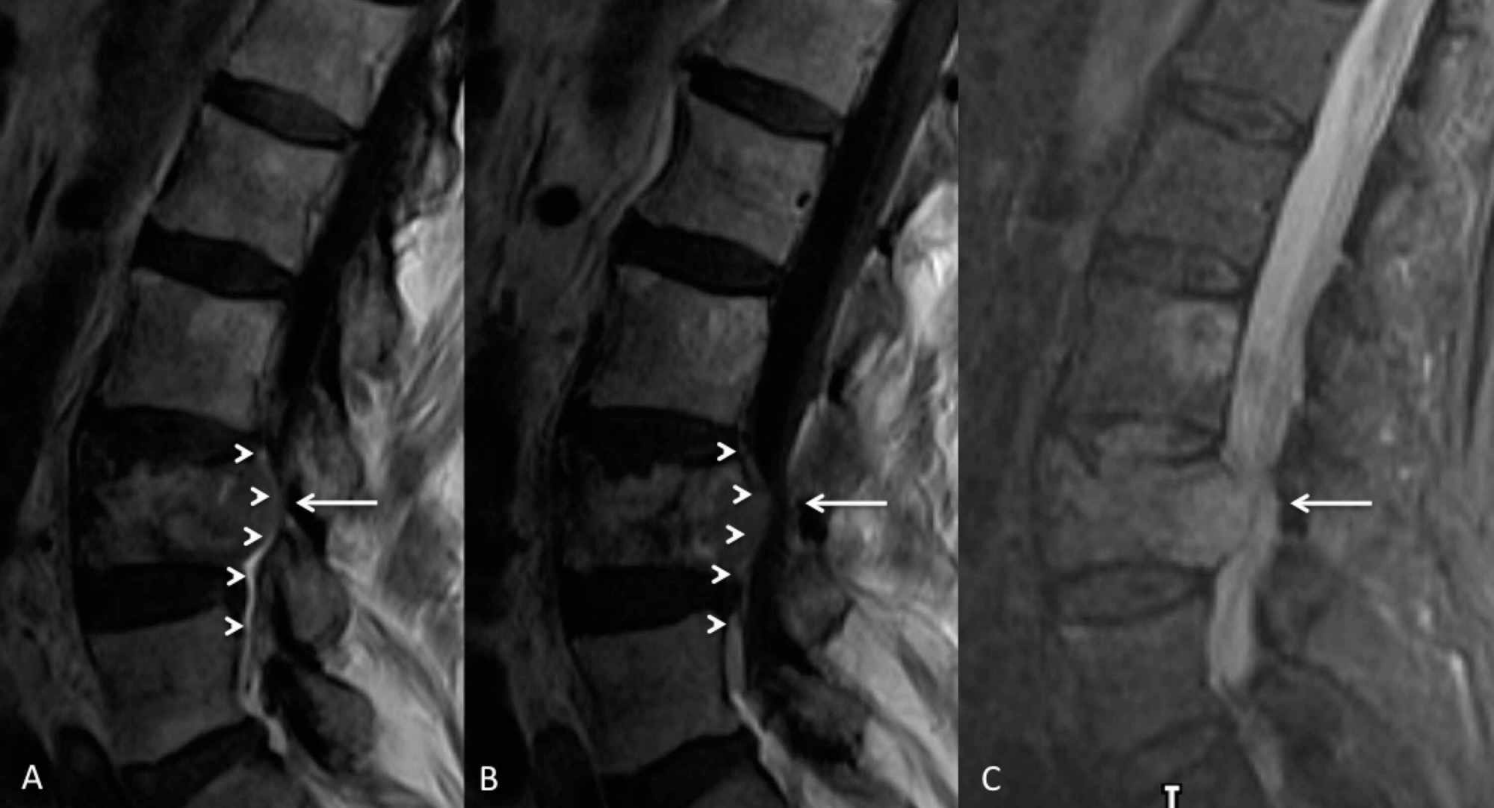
Pre-treatment parasagittal contrast-enhanced T1-weighted image (A), T1-weighted (B) and STIR-weighted (C) acquisitions demonstrating an expansile metastasis within the diffusely infiltrated L4 vertebral body and epidural metastatic disease (arrow heads) which result in moderately severe central canal stenosis (white arrow). STIR, short TI inversion recovery
